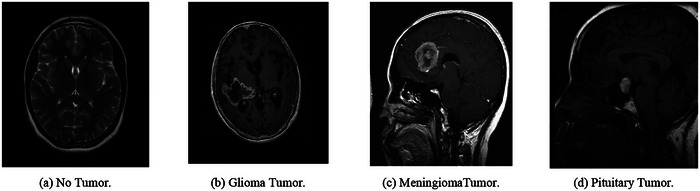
MRI of different brain tumor classes.
